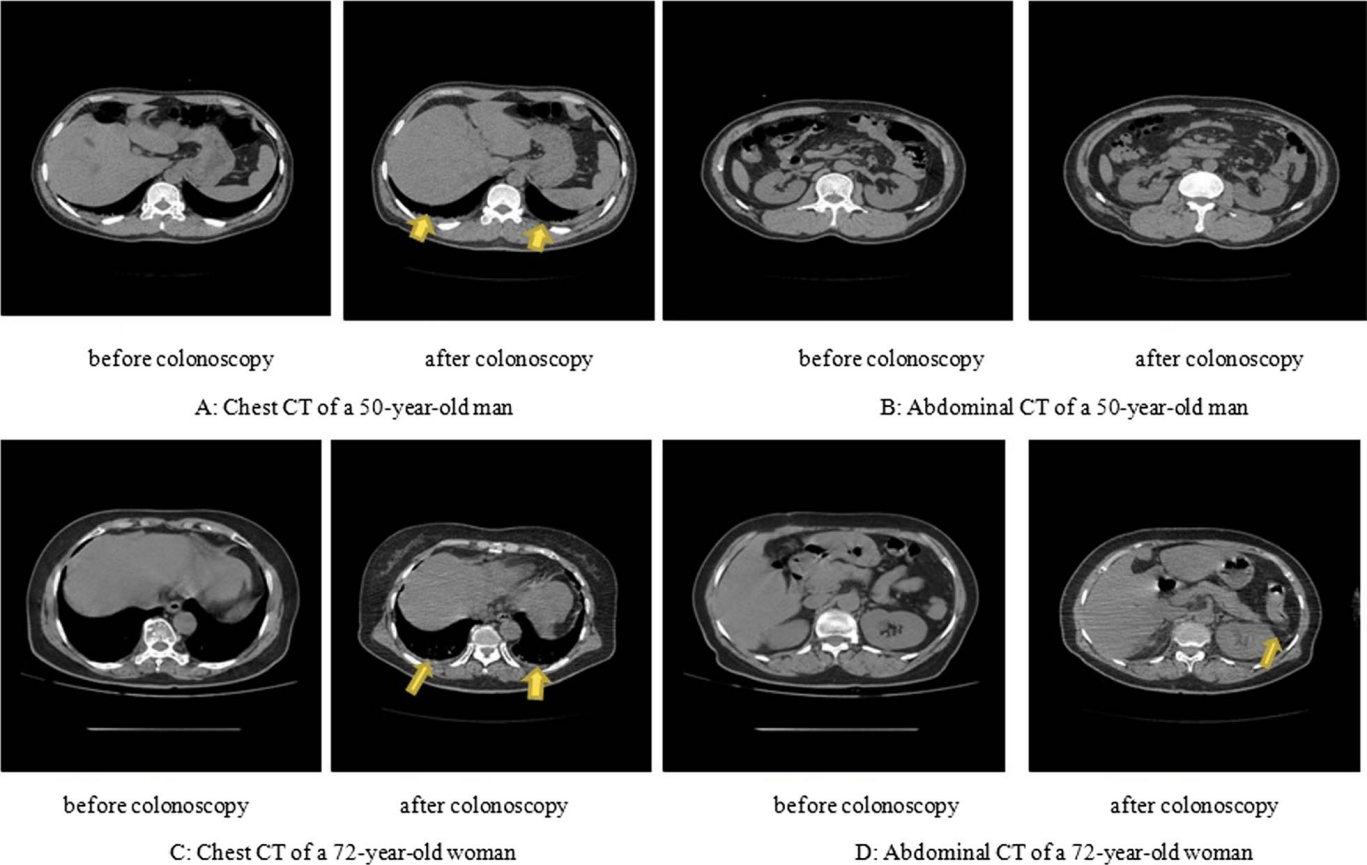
chest and abdominal CT before and after colonoscopy. A Chest CT of a 50-year-old man. Normal CT before colonoscopy, but abnormal CT with pleural effusion after colonoscopy. B Abdominal CT of a 50-year-old man. Normal CT before and after colonoscopy. C Chest CT of a 72-year-old woman. Normal CT before colonoscopy, but abnormal CT with pleural effusion after colonoscopy. D Abdominal CT of a 72-year-old woman. Normal CT before colonoscopy, but abnormal CT with exudation in pancreas tail after colonoscopy
